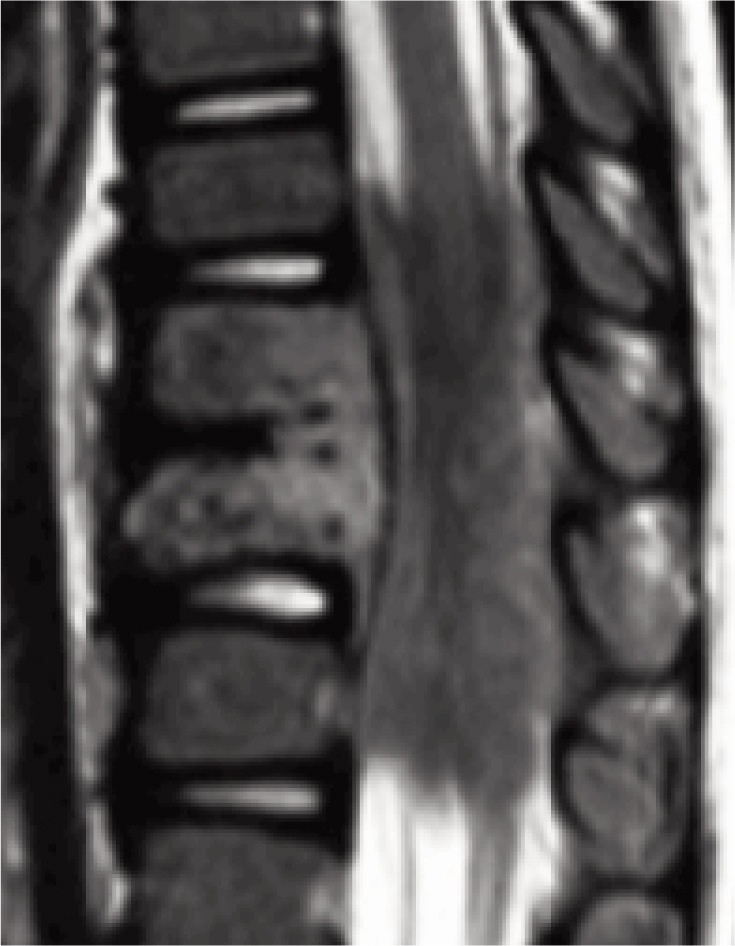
Enlarged T2 weighted MRI from T9 to T12.
